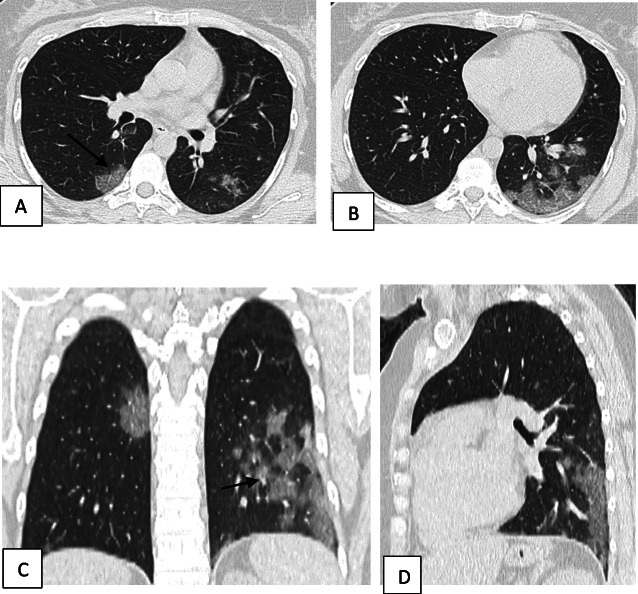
Unenhanced chest CT of a 32-year-old female patient with COVID-19. a, b Axial lung window images show bilateral subpleural and broncho-vascular areas of crazy paving [arrows]. c Coronal and d sagittal reformatted images. CT score is 7
